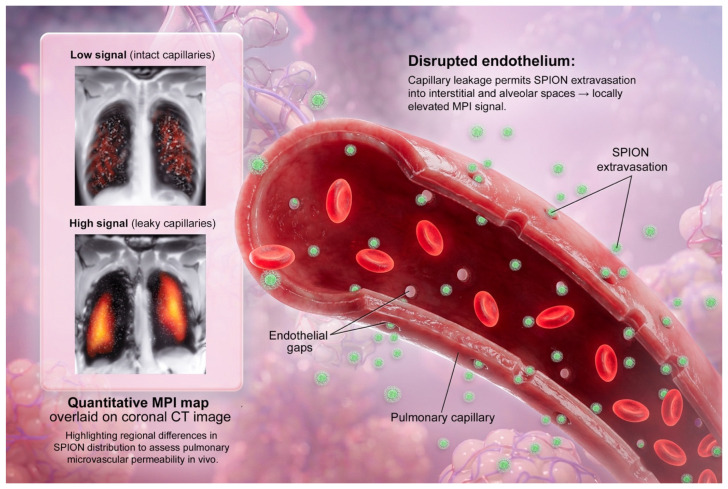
Pulmonary vascular permeability visualized using MPI, showing low signal in intact capillaries and elevated signal with SPION extravasation due to endothelial disruption; the quantitative MPI-CT overlay is an artist’s rendering.
